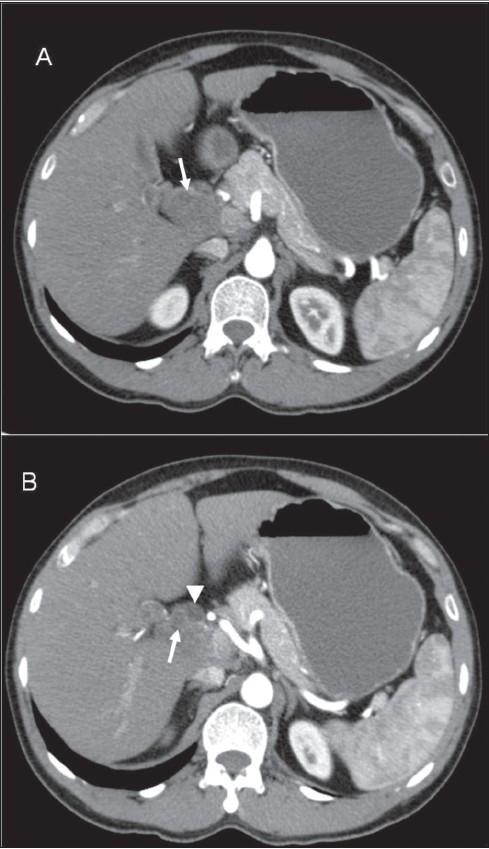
Axial contrast-enhanced CT scans show a heterogeneous soft-tissue lesion (arrow) in the porta hepatis with a dilated common bile duct (arrow head)
